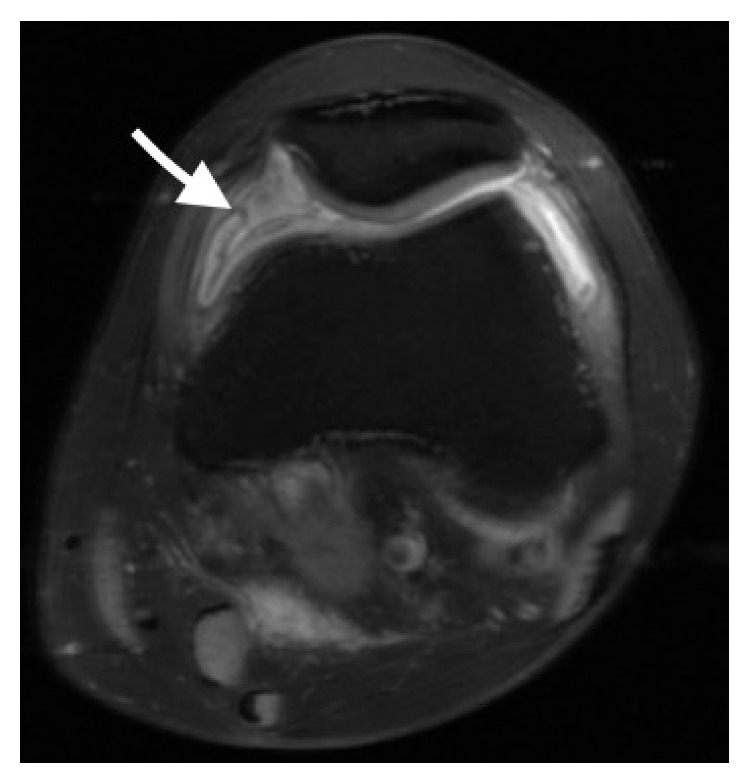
MRI (axial view) of left knee showing synovial thickening (arrow).
